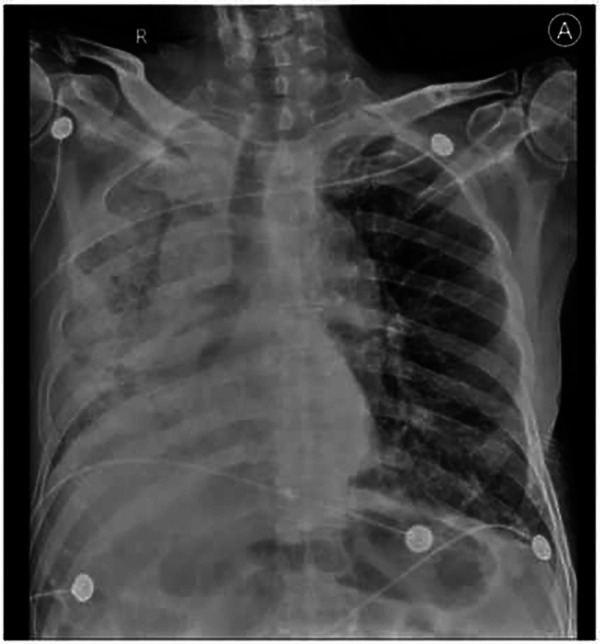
Clinical imaging of a 62-year-old man diagnosed with IPF before LTx and who underwent single-LTx surgery 2 years before COVID-19. Chest radiograph image shows massive consolidation of the allograft (right lung). IPF, idiopathic pulmonary fibrosis.
